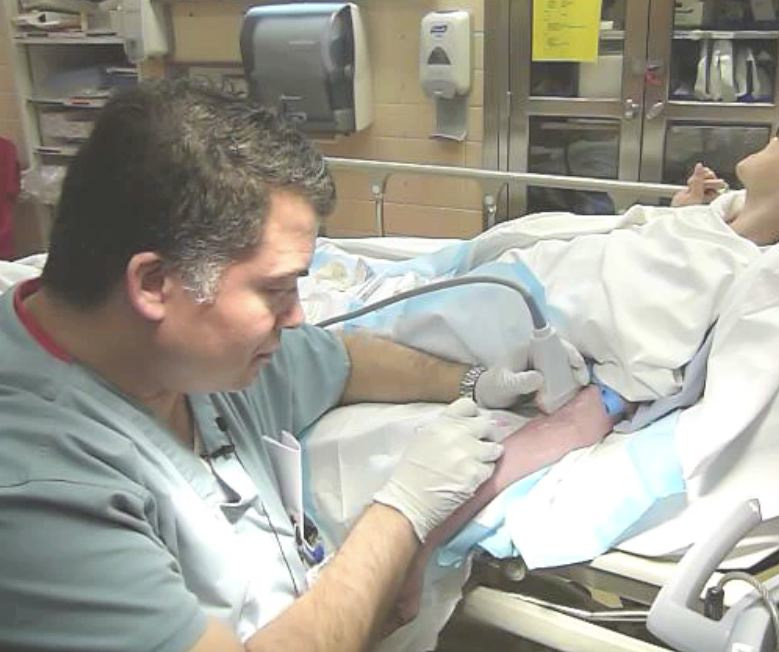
Dr, Riley performing an ultrasound guided long-axis antecubital intravenous line placement.
